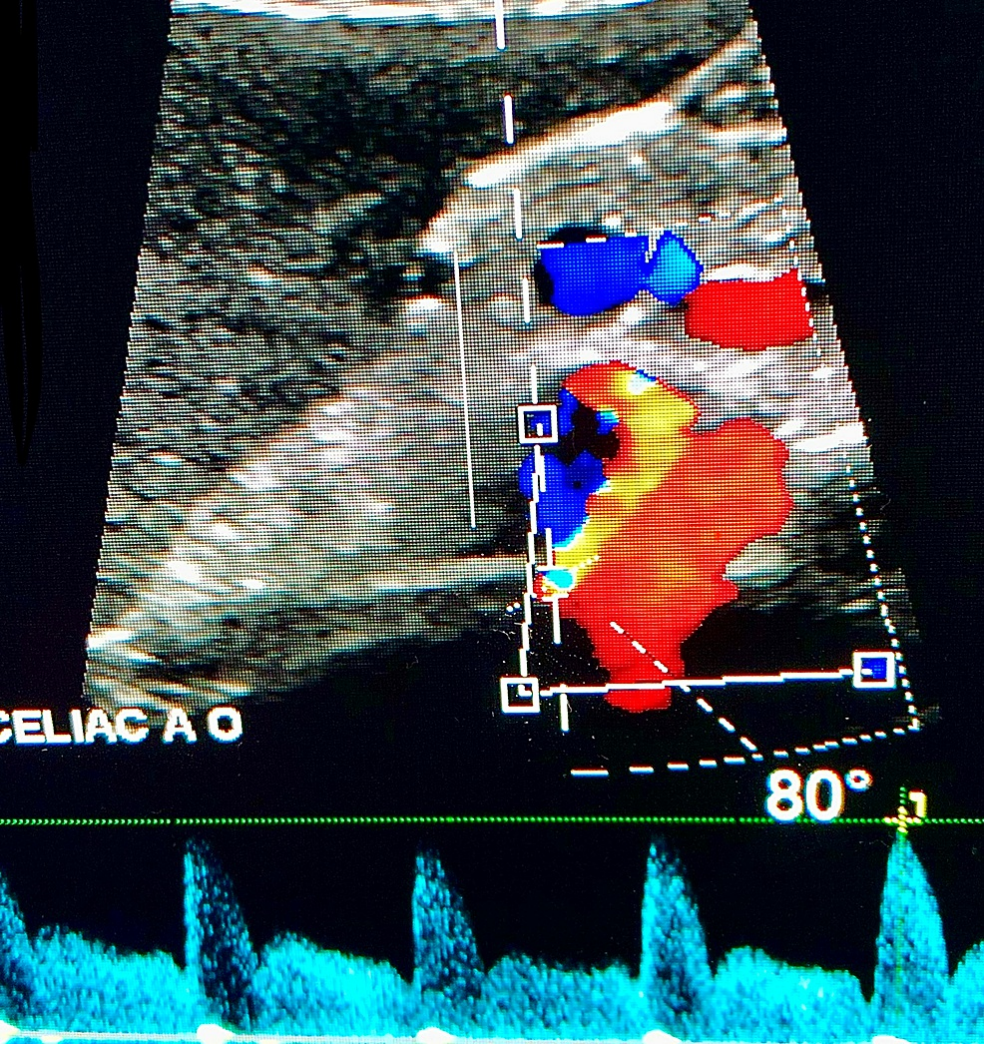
Mesenteric ultrasound showing the angle between the celiac artery and aorta on deep inspiration.
